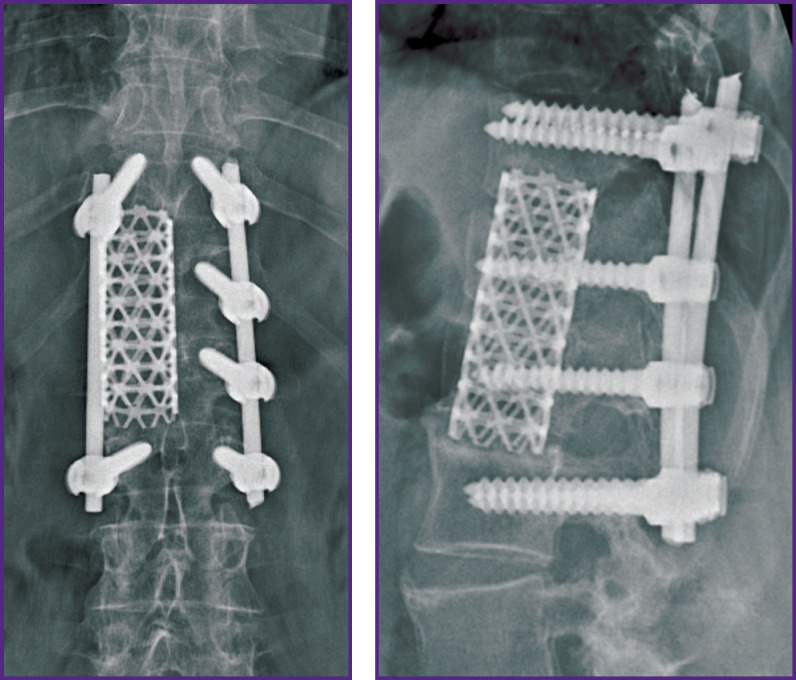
X-ray pictures of thoracolumbar transitional spine of patient I. after the second stage of spondylosynthesis
